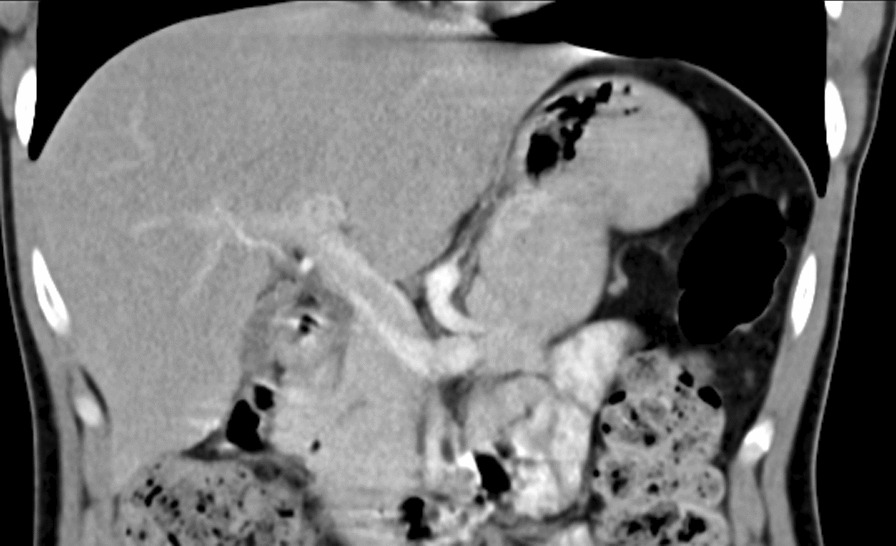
The contrast-enhanced computed tomography scan of the abdomen, which shows portal vein thrombosis
